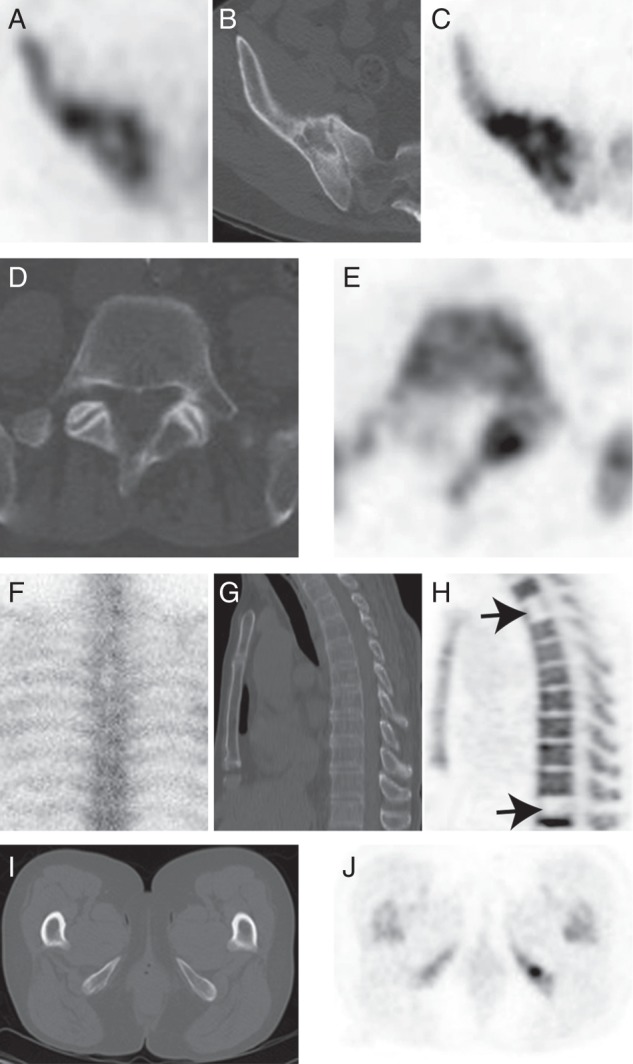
(A–C) Lesion in the right ilium detected by all three modalities: (A) axial ^99m^Tc-MDP SPECT; (B) axial CT; (C) axial ^18^F-NaF PET. (D and E) Example of ^18^F-NaF PET signal emphasizing a CT abnormality in the left posterior elements of the L5 vertebral body: (D) axial CT; (E) ^18^F-NaF PET. (F–J) Examples of lesions only identified on ^18^F-NaF PET/CT. Vertebral metastases: (F) ^99m^Tc-MDP bone scintigraphy coronal image. (G) CT sagittal reconstruction. (H) ^18^F-NaF PET sagittal reconstruction showing metastases with low tracer uptake (arrows). Metastasis in the inferior pubic ramus: (I) axial CT image; (J) ^18^F-NaF PET image.
